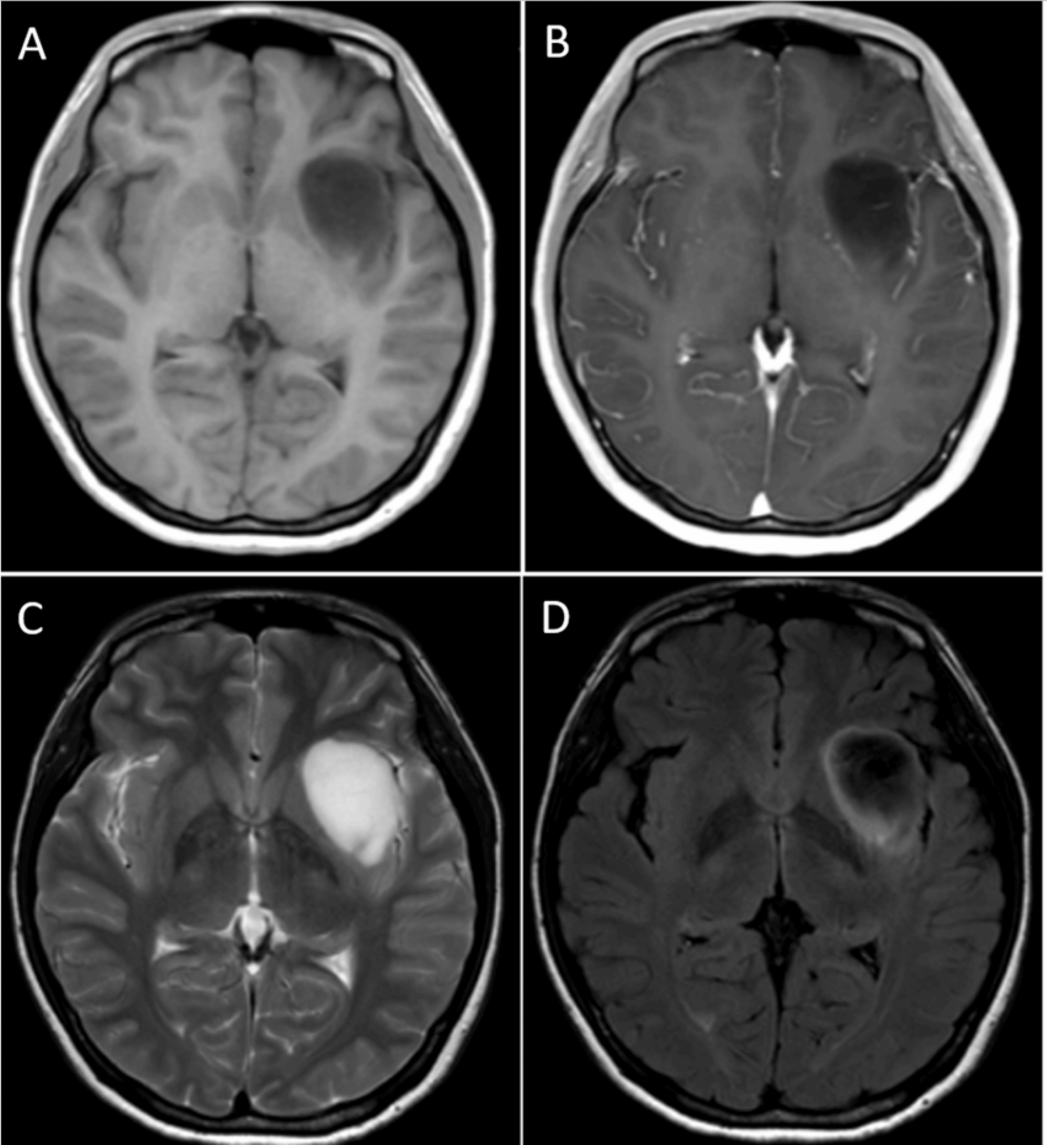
Preoperative MRI demonstrating a left diffuse insular glioma. The T1-weighted image (A) and T1 contrast-enhanced image (B) show an enhancing rim surrounding the lesion. The T2-weighted image (C) and FLAIR image (D) exhibit a T2-FLAIR mismatch sign, suggestive of an IDH-mutant astrocytoma. IDH: isocitrate dehydrogenase; FLAIR: Fluid-Attenuated Inversion Recovery
